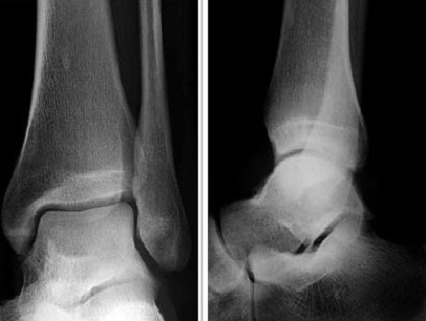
Radiographies standards de la cheville gauche (préopératoire): normale
